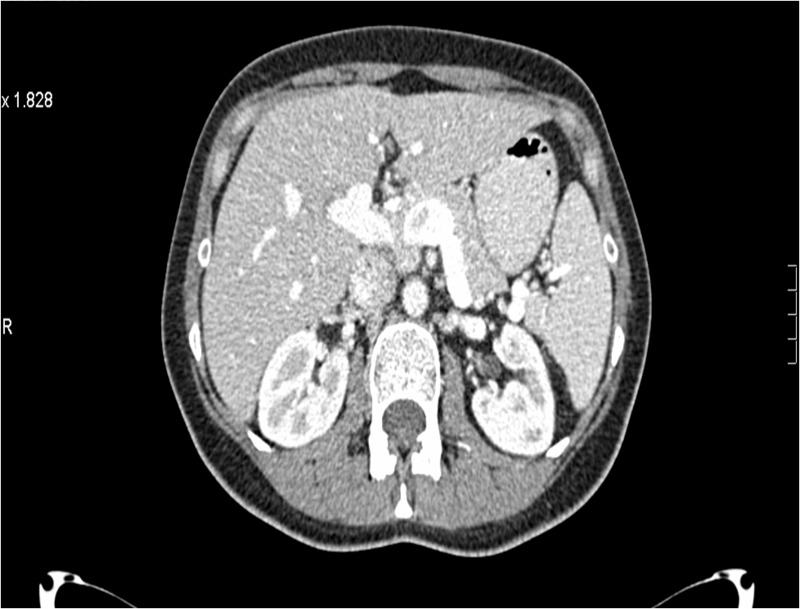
Patient 1: Post-treatment CT shows resolution of liver lesion
